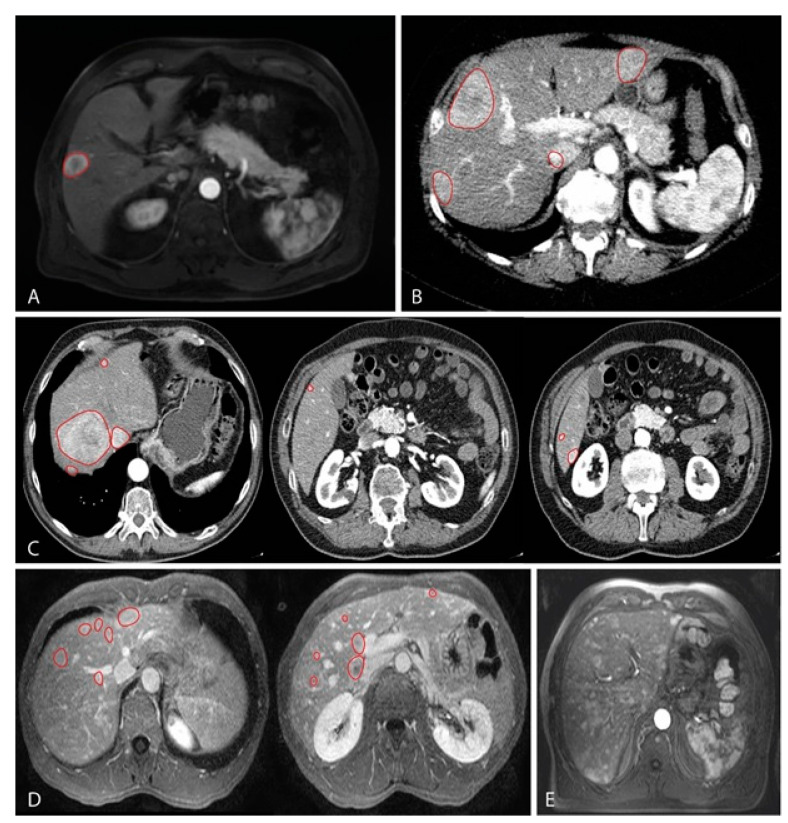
MRI images representing the classification based on the number of lesions found in metastatic UM in the liver, which have been contrasted by red markings; (A) T1 weighted MRI with contrast showing a single nodular lesion in the liver; (B) T2 weighted MRI with contrast showing four lesions; (C) T2 weighted MRI sequence with contrast showing a total of seven lesions. Images from left to right correspond, respectively, from cranial to caudal localization; (D) T2 weighted MRI sequence with contrast showing a total of 12 lesions. Images from left to right correspond, respectively, from cranial to caudal localization; (E) T2 weighted MRI with contrast showing innumerable widespread small lesions throughout all liver segments. No red markings are present due to the number of lesions.
